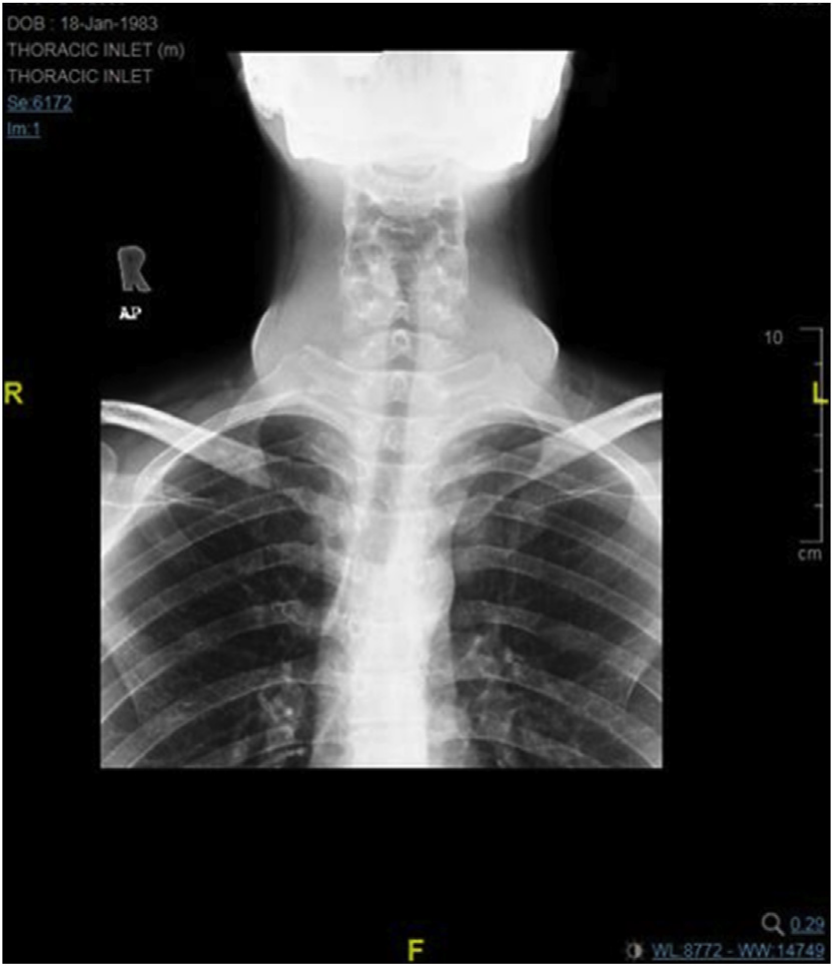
Chest X-Ray (Antero-posterior view) showed diffuse swelling in the neck with patent none deviated trachea.
